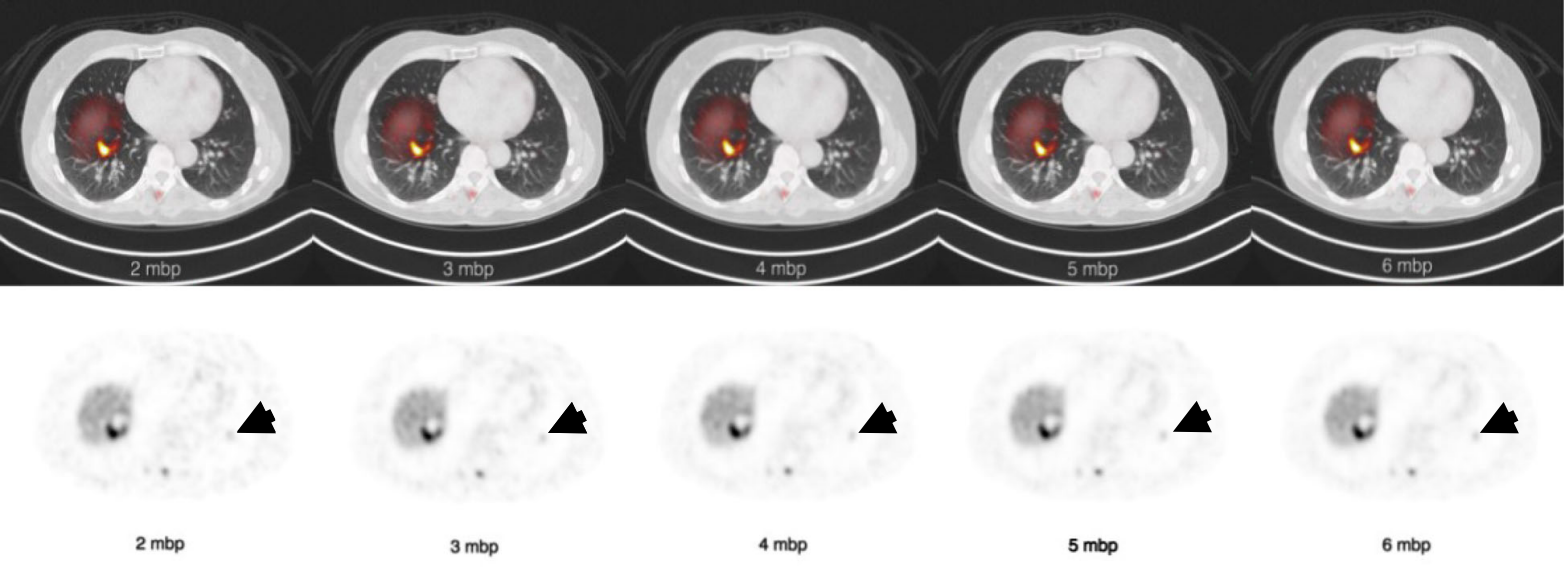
Example of transversal PET/CT fusion and PET only images. The images show multiple lesions including one lesion in the liver and one lesion with low uptake in the left basal lung (arrow). The lung lesion was detected on 3-6 mbp and was missed on 2 mbp. All other lesions were detected on all mbps
